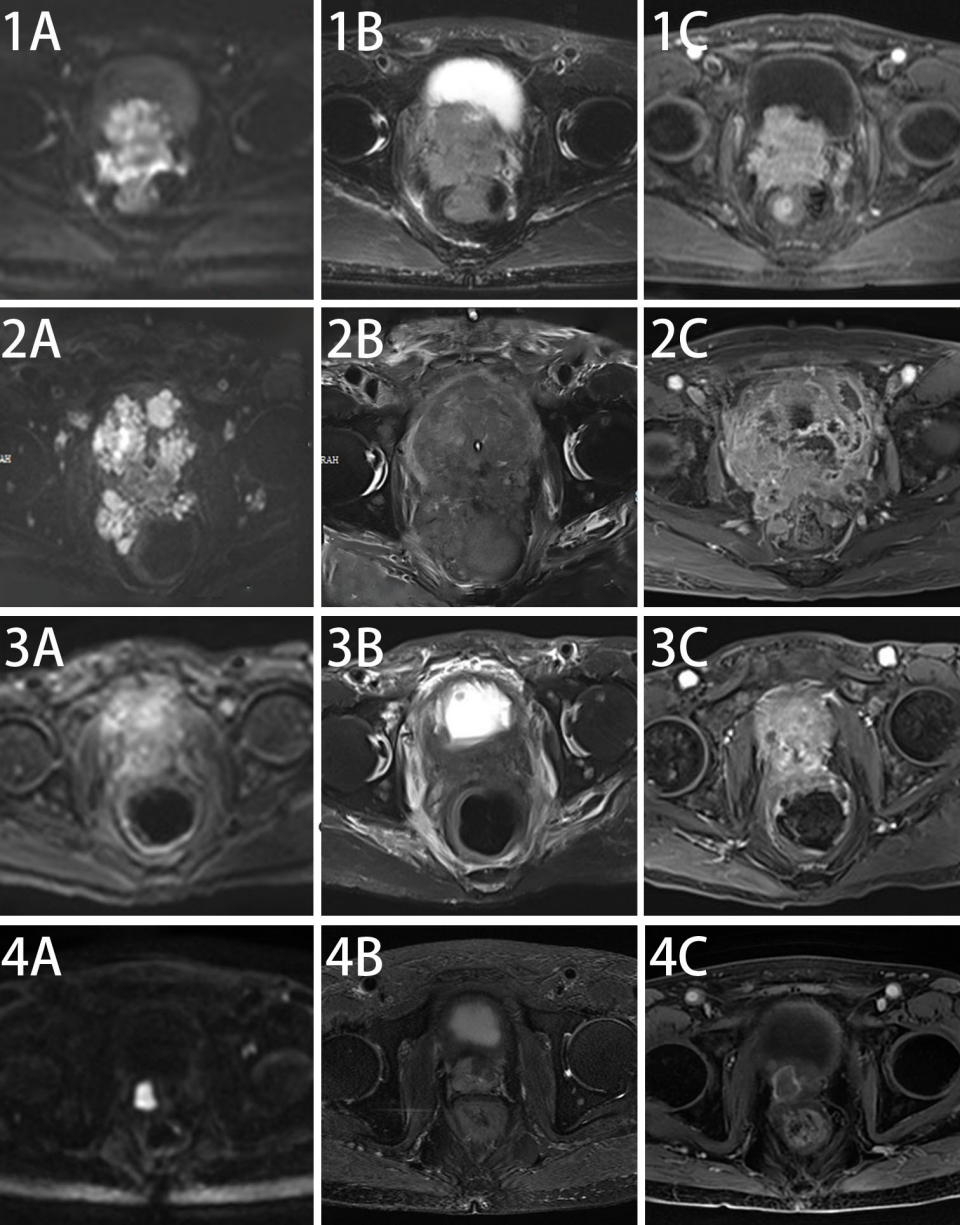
Changes in pelvic MRI images before and after treatment in this patient. Pelvic MRI Scan at diagnosis of this disease (1A–C), after chemotherapy (2A–C), after five cycles of anlotinib + tislelizumab treatment (3A–C) and maintenance treatment of anlotinib + tislelizumab (4A–C).
